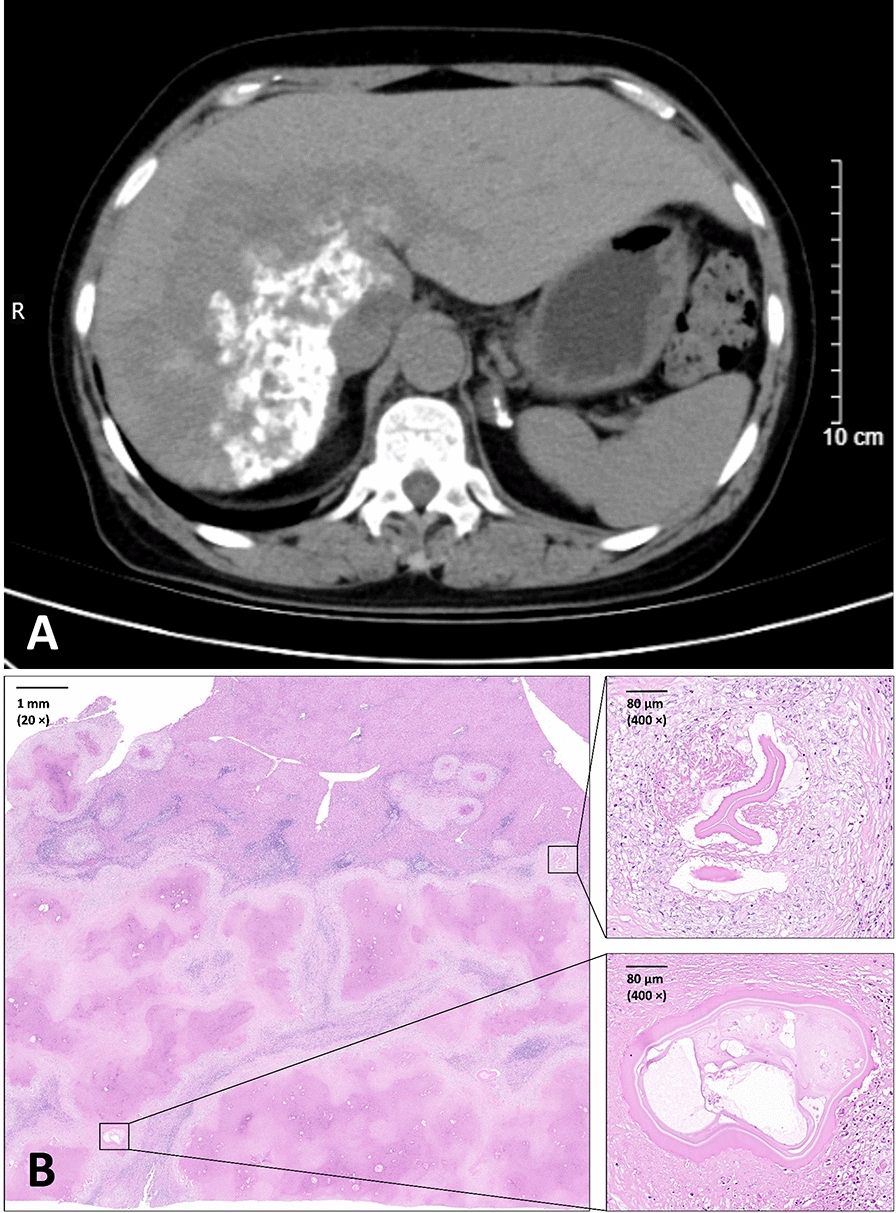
Computed tomography images and pathological observations of lesion
